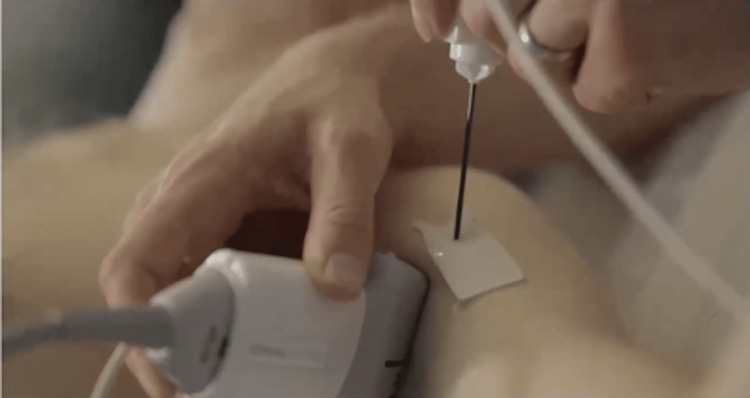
NeedleTrainer Device Image from Ultrasound I. NeedleTrainer - Intelligent Ultrasound [6] NeedleTrainer: Intelligent Ultrasound, Cardiff, UK
